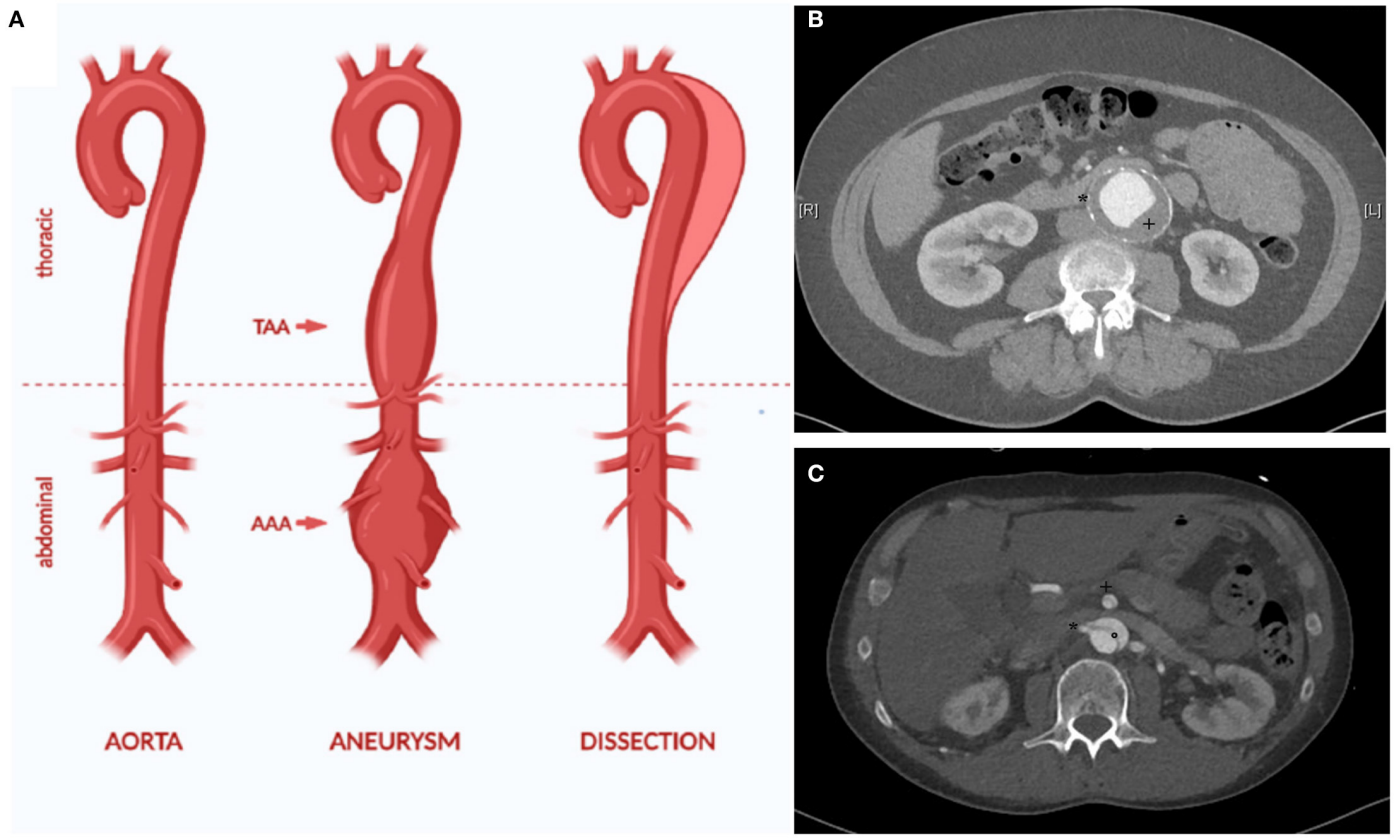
The aorta can be affected by different disease, for example aortic aneurysm and dissection (A). AA is defined as dilatation of all layers of the vessel wall (true aneurysm). This can affect all parts of the aorta, for example the thoracic and the abdominal aorta (thoarcic aortic aneurysm (TAA) and abdominal aortic aneurysm (AAA). The CT angiography depicts characteristic traits of an infrarenal aneurysm with thrombus (+) and calcification of the aortic wall (^*^) (B). In AD, an initial intima lesion causes separation of the aorta in a true and false lumen by a dissection membrane (°) (C). Visceral arteries such as the superior mesenteric artery (+) and the right renal artery (^*^) can be affected by the dissection causing organ ischemia. Due to the reduced stability of the aortic wall, most patients with AD require surgical treatment in the long term due to chronic dilatation of the aorta [modified from (2)].
